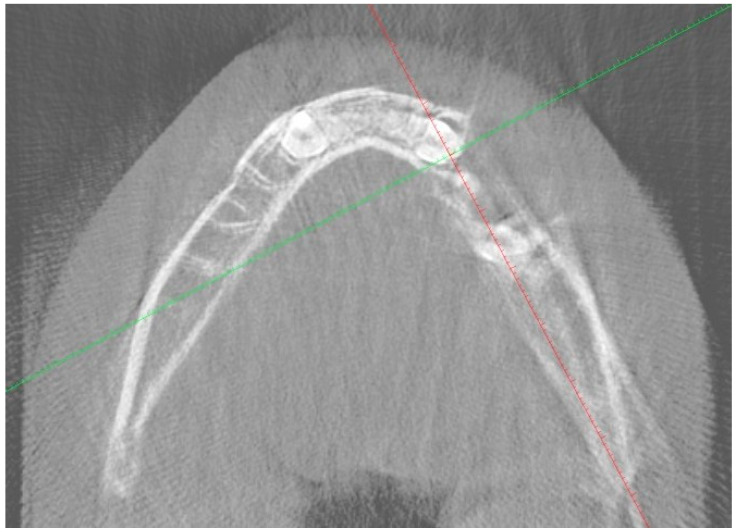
CBCT image in horizontal plane.
